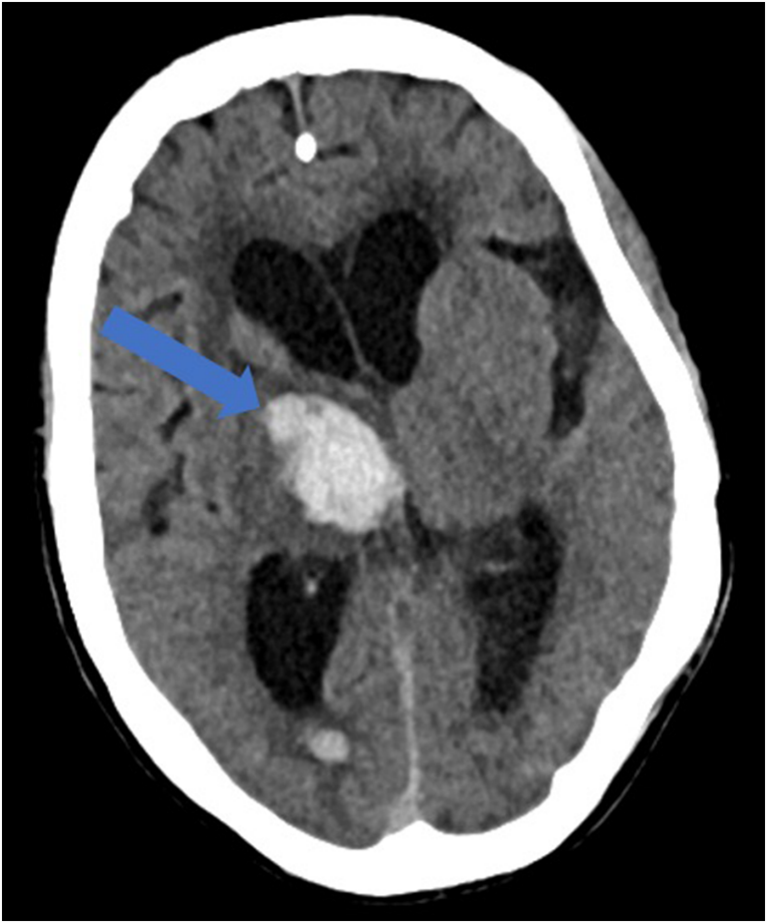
Intracranial hemorrhage in a 60-year-old: Axial non-contrast CT scan demonstrates intraparenchymal hemorrhage within the right basal ganglia and thalamus, with associated intraventricular extension, dilatation, and midline shift (blue arrow).
